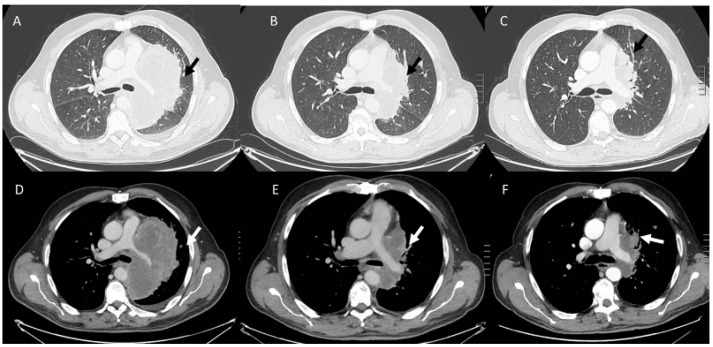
Partial response pattern in non small lung cancer (NSLC) treated with immunotherapy (arrows). CT scan assessment in pre-treatment phase (A,D), at 3 months (B,E), and after 6 months (C,F).
